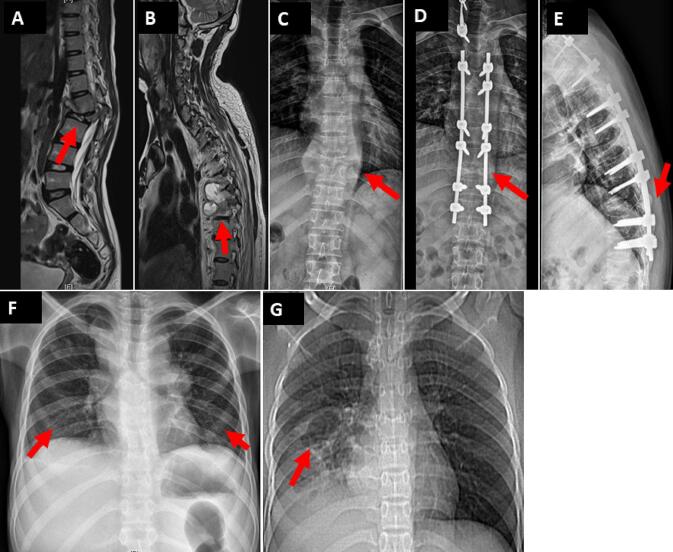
(A) Sagittal T2-weighted Magnetic resonance imaging (MRI) spine without contrast of 9-year-old female (case 1) showing near complete destruction of L2 (Lumbar) vertebra with pre- and paravertebral collection with bilateral psoas collection. (B) Sagittal T2-weighted MRI spine with contrast of 47-year-old male (case 2) showing interval increase in the destruction of T6-T7 (Thoracic) vertebral bodies, increase in extent of prevertebral, paravertebral, epidural and anterior subligamentous extension. (C) Thoracic spine anteroposterior (AP) view of 24-year-old male (case 4) showing paravertebral abscess at the level of T10 vertebra requiring decompression and stabilization. (D) Case 4 thoracic spine AP view post-surgical correction. (E) Case 4 thoracic spine lateral view post-surgical correction. (F) Chest radiography AP view of 16-year-old female (Case 6) shows bilateral lung nodular consolidation. (G) Computed Tomography (CT) of thorax without contrast of 16-year-old female (Case 8) shows consolidation with cystic changes of entire right lower lobe with branching nodular opacities in superior basal segment of right lower lobe.
